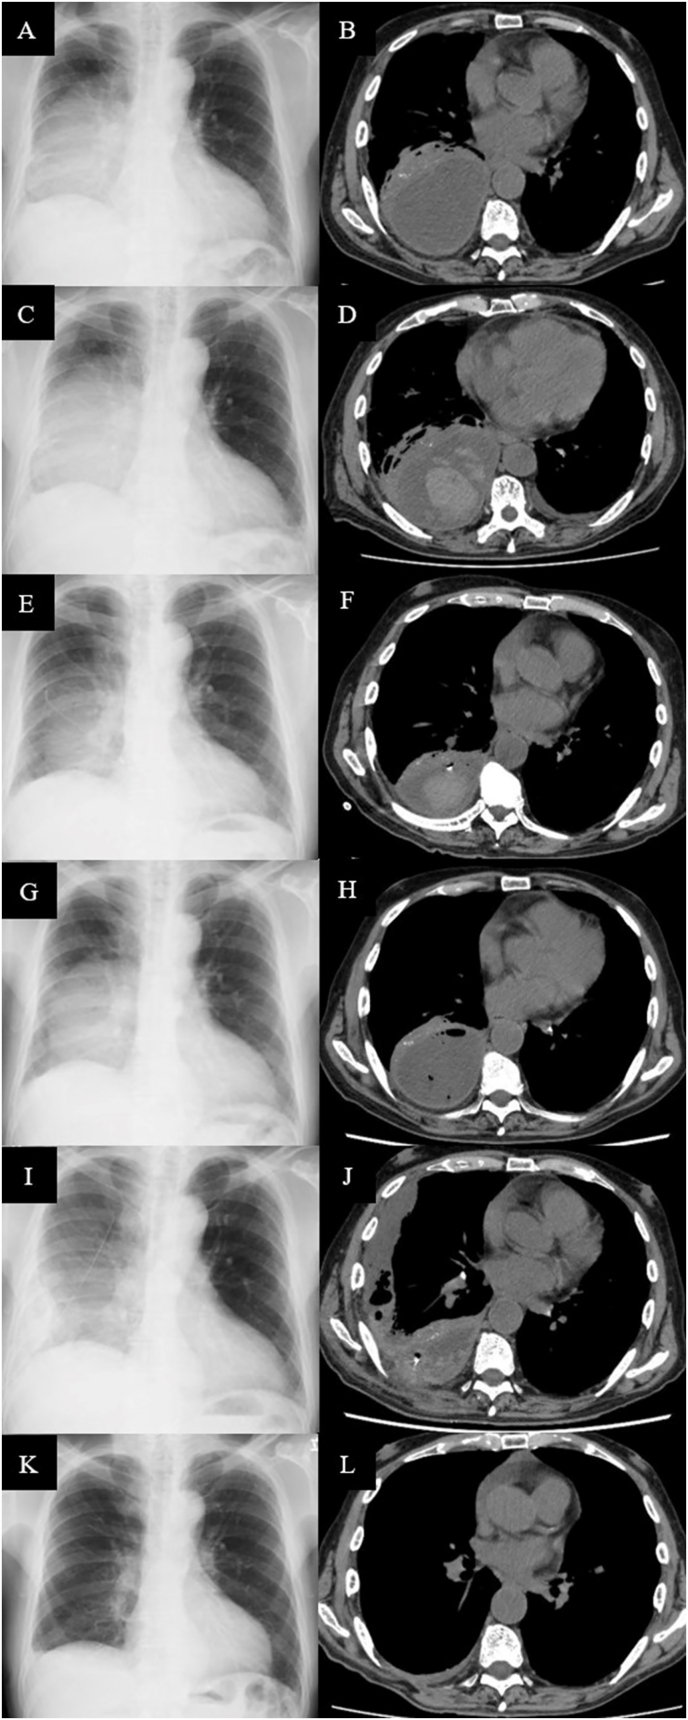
Imaging results of a 77-year-old man with *Actinomyces* empyema. On admission, chest X-ray revealed opacities in the right middle and lower lung fields (A), and computed tomography (CT) scan showed a loculated pleural effusion in the right lung (B). On day 4, chest X-ray displayed persistent opacities in the right middle and lower lung fields (C). CT imaging revealed a high-density area suggestive of hemorrhage, leading to the decision to withhold urokinase administration (D). Following antibiotic therapy and drainage, chest X-ray showed improvement in opacities (E), and CT scan revealed a reduction in pus accumulation (F). However, after the patient developed fever on day 17, chest X-ray showed increased opacities in the right lung (G), and CT scan indicated worsening pleural effusion (H). Following video-assisted thoracoscopic surgery (VATS), chest X-ray demonstrated improvement in the right lung opacities (I), and CT scan confirmed further reduction in pus accumulation (J). Complete resolution of the empyema was confirmed after an 11-month course of antibiotics (K, L).
